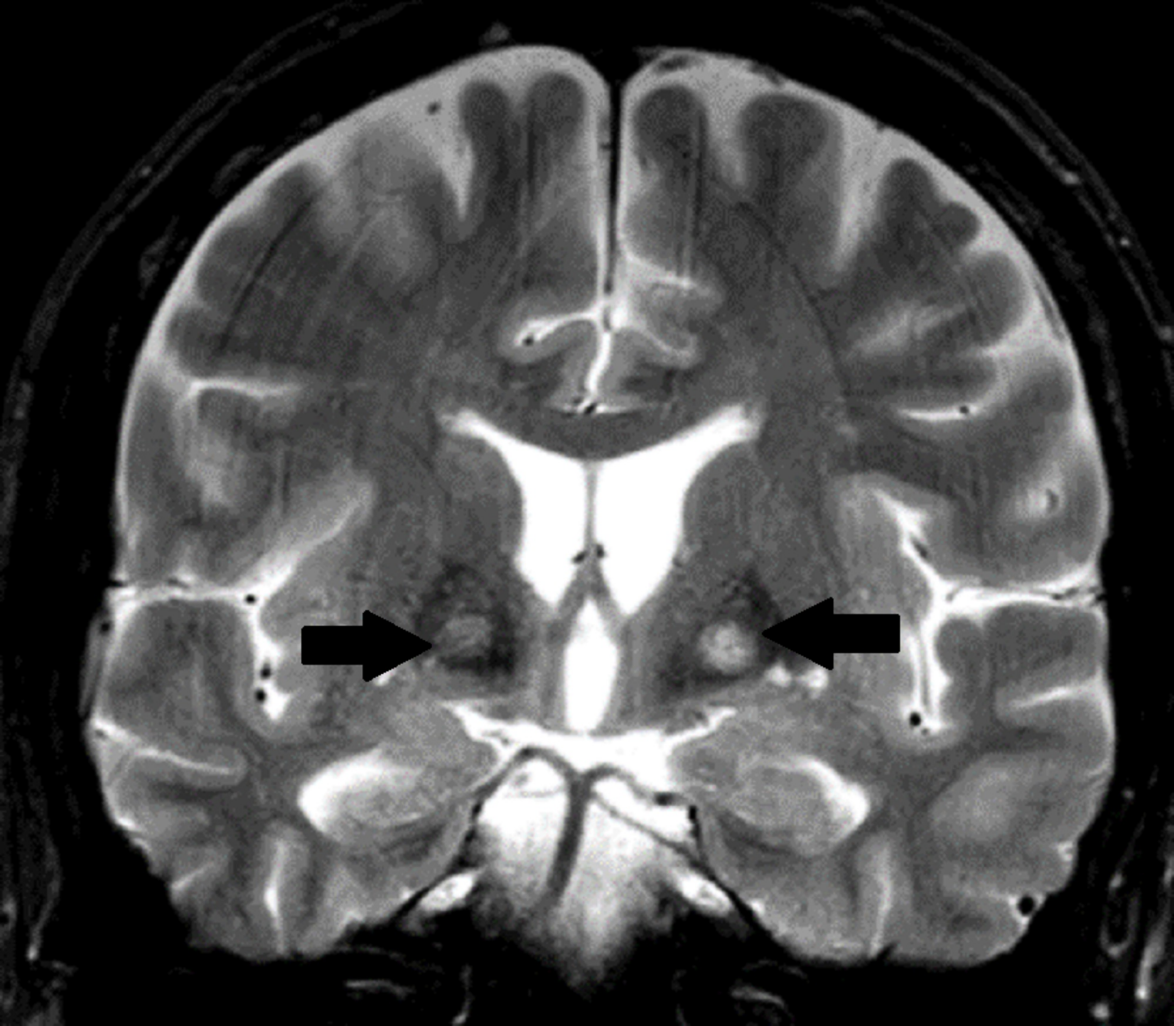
MRI FLAIR Coronal FLAIR sequence on the MRI showed symmetrically decreased T2 signal within the basal ganglia with an area of increased signal intensity (see arrows) suggestive of neutrophil necrosis. FLAIR: fluid-attenuated inversion recovery.
